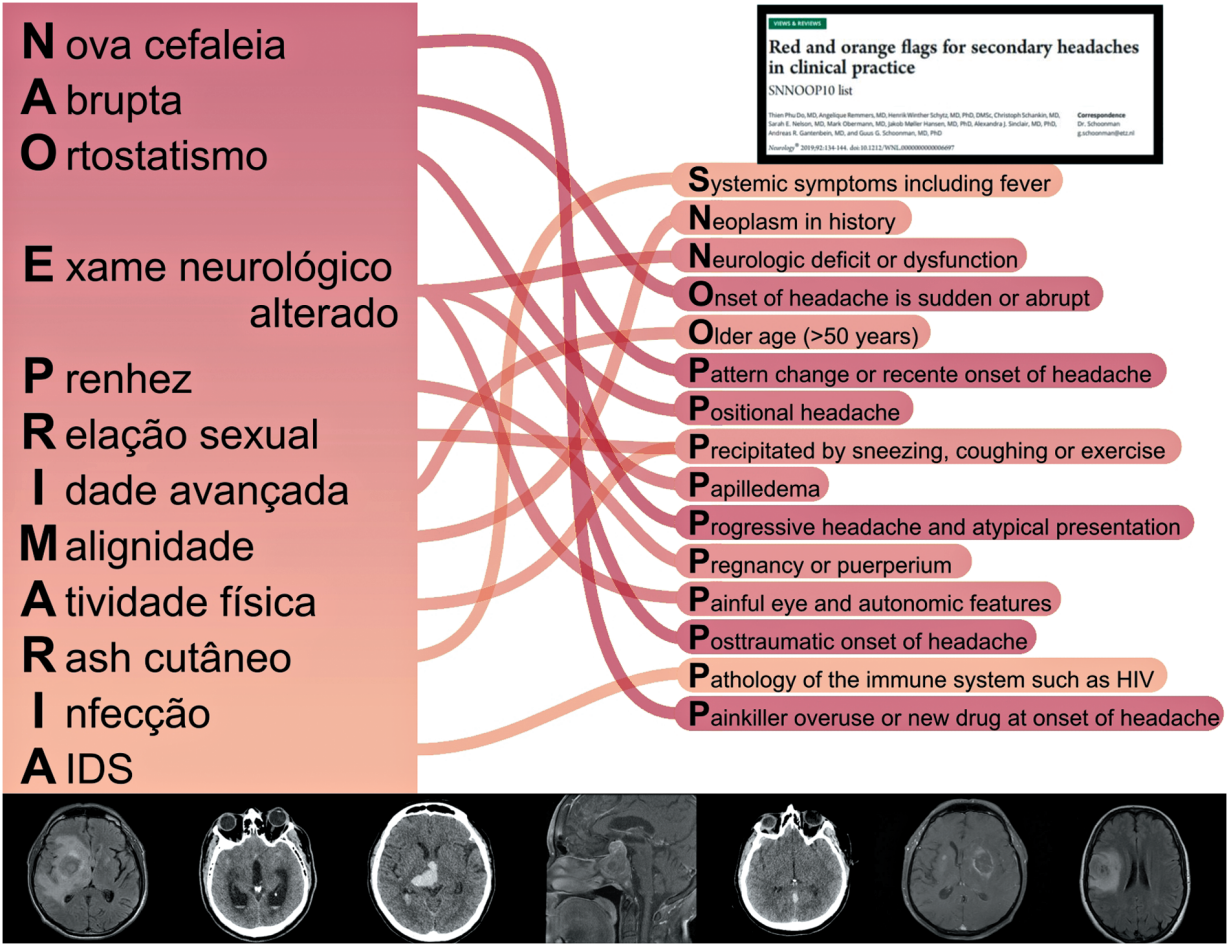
Notes: On the left side, “NÃO É PRIMÁRIA” triggers linked to their SNNOOP10 red flags, on the right side. Below, computed tomography (CT) and magnetic resonance imaging (MRI) scans of examples of secondary etiologies.
